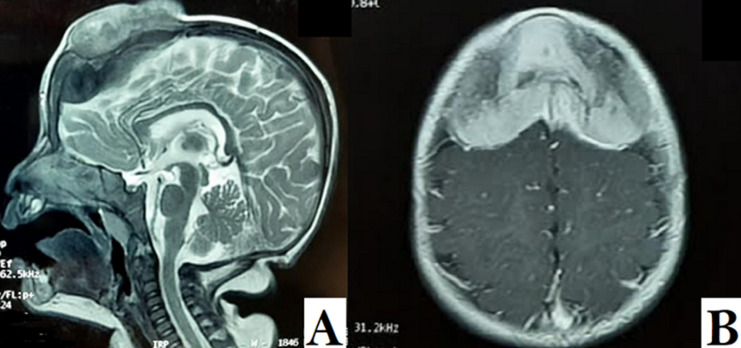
control cerebral magnetic resonance imaging of the patient at 6 months follow-up
